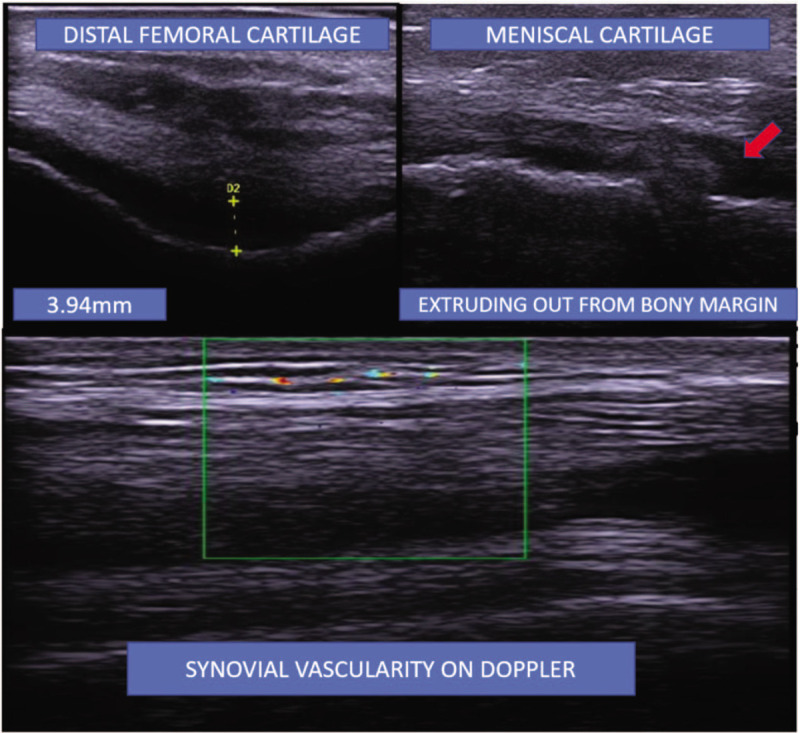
Ultrasonographic evaluation of knee at the baseline. Distal femoral cartilage measured at the mid-point of lateral and medial condyles in the position of knee being maximally flexed.
